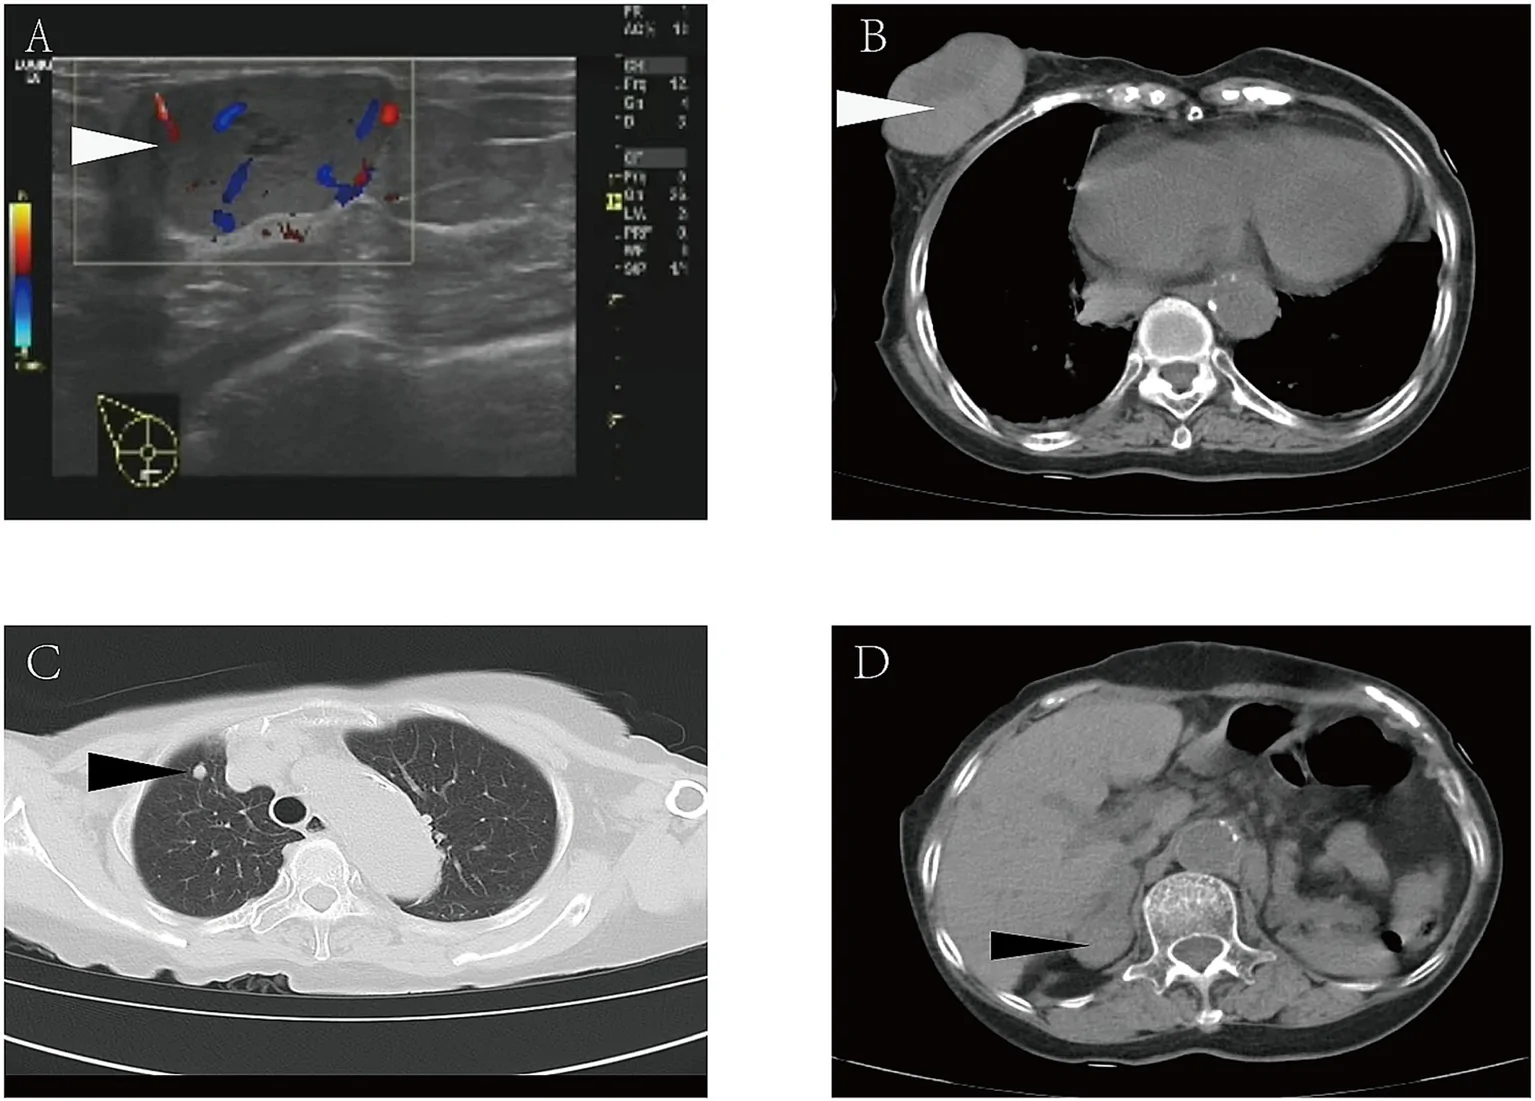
Imaging findings of the breast mass and suspected lesions (indicated by arrows). (A) Ultrasound 2 years before admission: 2.5 × 1.5 cm hypoechoic nodule with clear margins, BI-RADS 3. (B) Non-contrast CT at admission: 6.5 × 6 × 7.5 cm right breast mass with mixed solid-cystic components, suggesting intratumoral hemorrhage. (C) CT: Right pulmonary nodule. (D) CT: Right adrenal mass.
